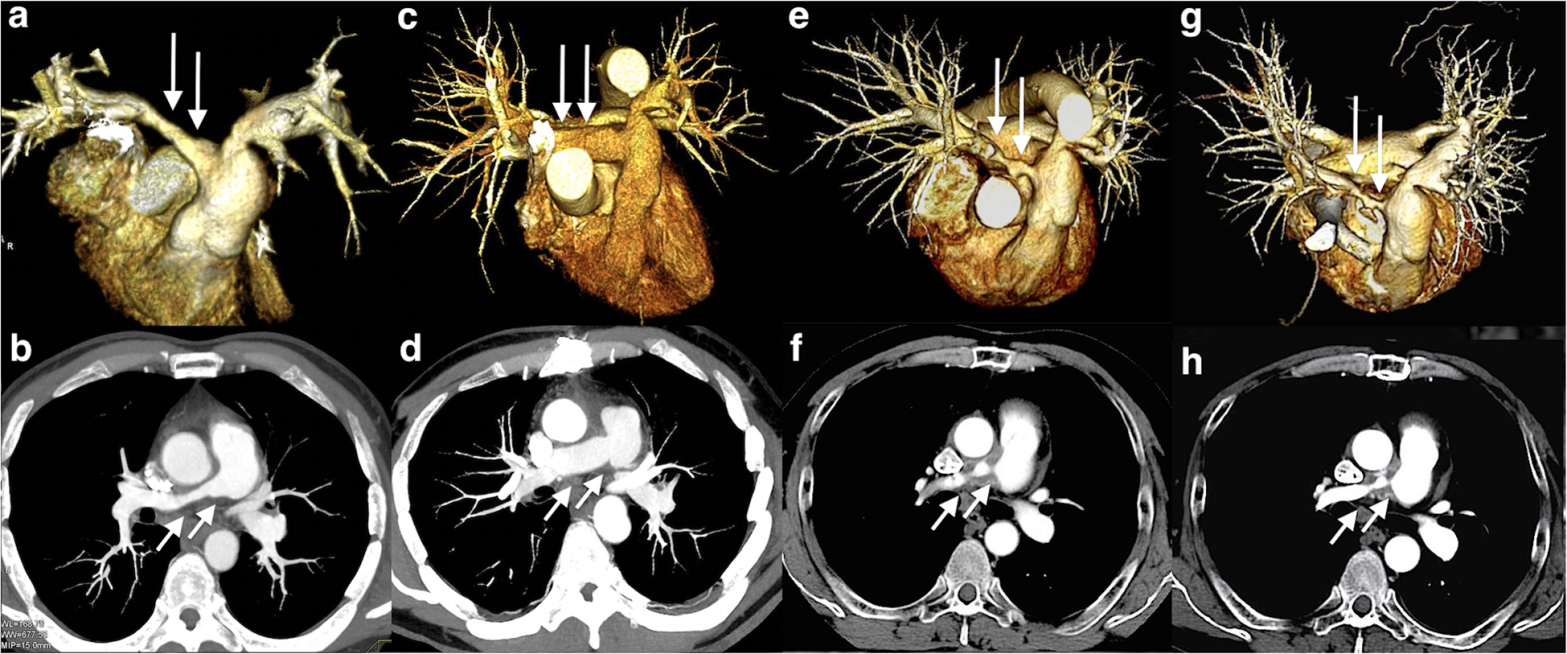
Chest CT images: (a, b) Before surgery, showing stenosis of the right PA (arrows); (c, d) At 6 months after surgery, showing the patent grafts of the right PA (arrows); (e, f) At 18 months after surgery, showing the stenosis of the right PA graft (arrows); (g, h) At 34 months after surgery, showing unchanged stenosis of the right PA graft (arrows)
